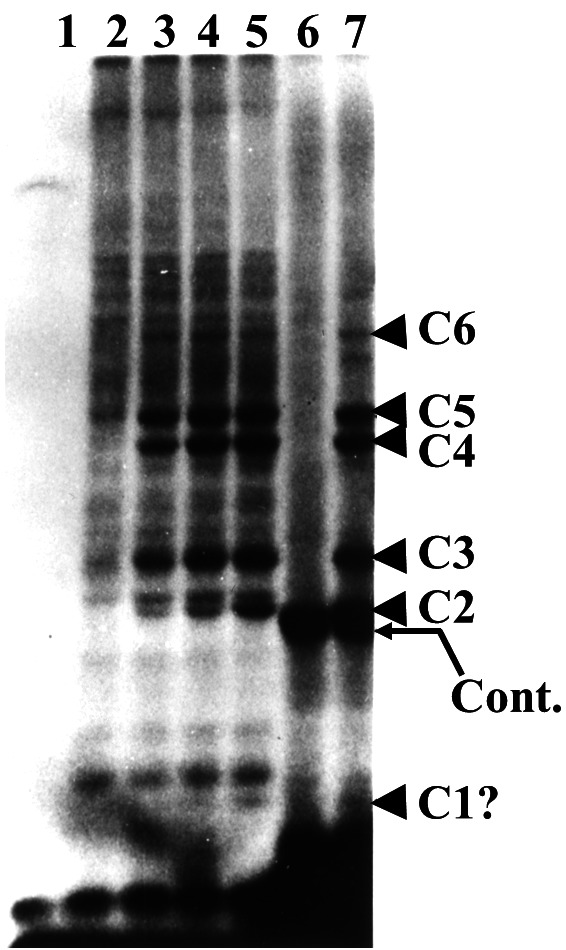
Multiple molecules of APF-1/ubiquitin are conjugated to the proteolytic substrate, probably signaling it for degradation. To interpret the data described in the experiment depicted in Figure 2 and to test the hypothesis that APF-1 is conjugated to the target proteolytic substrate, ^125^I-APF-1/ubiquitin was incubated along with crude fraction II (Figure 3 and text) in the absence (lane 1) or presence (lanes 2–5) of ATP and in the absence (lanes 1 and 2) or presence (lanes 3–5) of increasing concentrations of unlabeled lysozyme. Reaction mixtures resolved in lanes 6 and 7 were incubated in the absence (lane 6) or presence (lane 7) of ATP, and included unlabeled APF-1/ubiquitin and ^125^I-labeled lysozyme. C1–C6 denote specific APF-1/ubiquitin-lysozyme adducts in which the number of APF-1/ubiquitin moieties bound to the lysozyme moiety of the adduct is increasing, probably from 1 to 6. Reaction mixtures were resolved via sodium dodecyl sulfate-polyacrylamide gel electrophoresis (SDS-PAGE) and visualized following exposure to an X-ray film (autoradiography) (with permission from Proceedings of the National Academy of the USA; published originally in Hershko et al.^40^).
